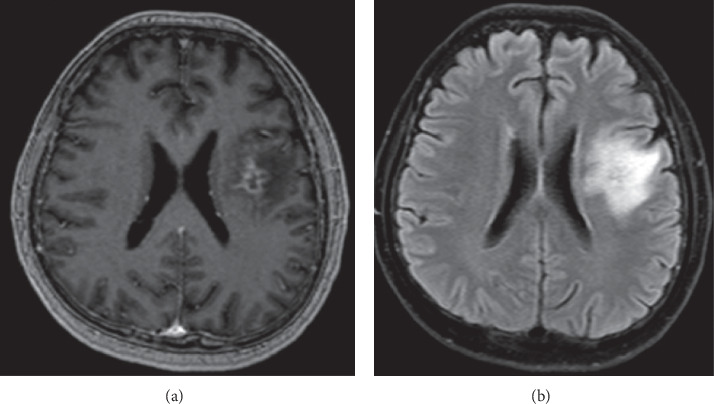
(a) Axial FLAIR v1. (b) Axial postcontrast T1 MPR. Patchy parenchymal enhancement predominantly involving the left subinsular region. The temporal operculum shows peripherally enhancing centrally cystic areas with restricted diffusion concerning for evolving meningoencephalitis with cerebral abscess formation.
